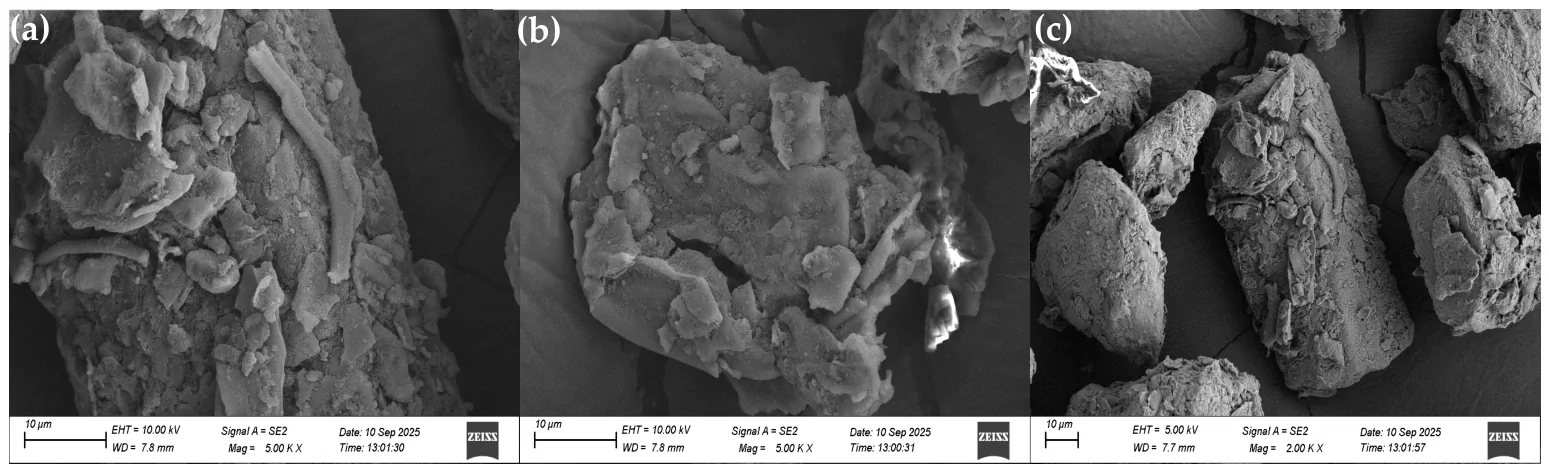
SEM images of turmeric root powder: (a) before extraction; (b) after HDES extraction without ultrasound treatment; (c) after ultrasound-assisted HDES extraction.
